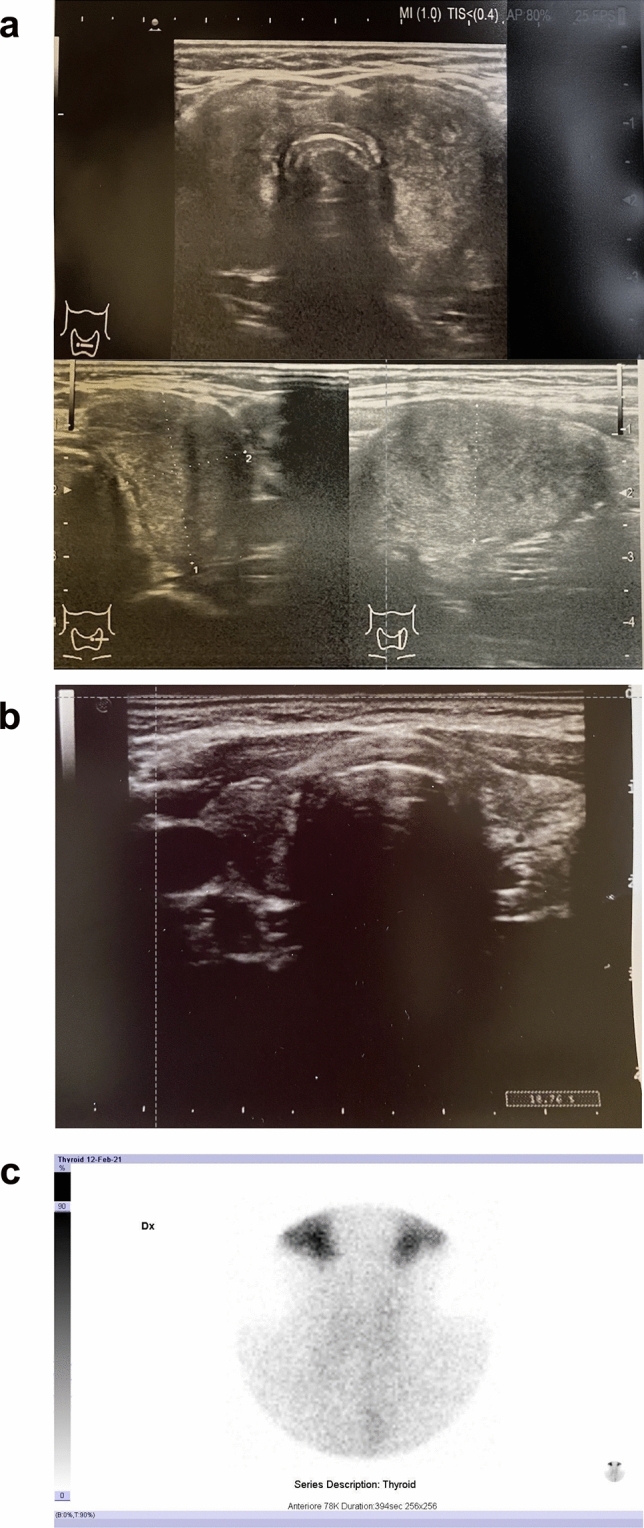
Thyroid ultrasound and scintigraphy in SARS-CoV-2 post-vaccination SAT. Ultrasound performed a at diagnosis and b at resolution of symptoms in a patient with subacute thyroiditis developed after mRNA vaccine for SARS-CoV-2 (Case 1, described in Table 1). At diagnosis thyroid was enlarged, highly inhomogeneous and nodular. After therapy, at resolution of symptoms, thyroid echostructure remained inhomogeneous, but thyroid volume was back to normal and there were no nodules. c Thyroid scintigraphy revealed low/absent thyroid uptake in a patient with subacute thyroiditis developed after mRNA vaccine for SARS-CoV-2 (Case 1, described in Table 1)
